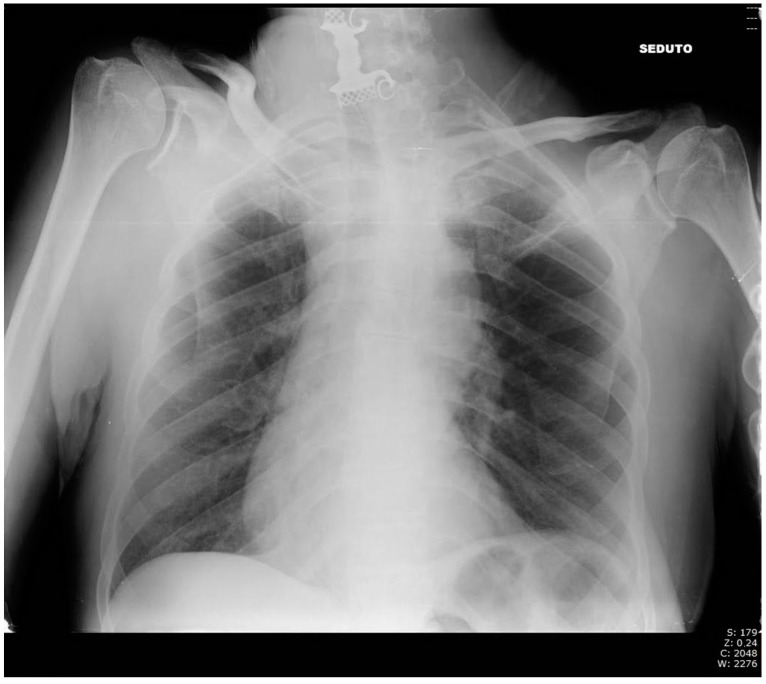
Chest radiography in the Emergency Room.
